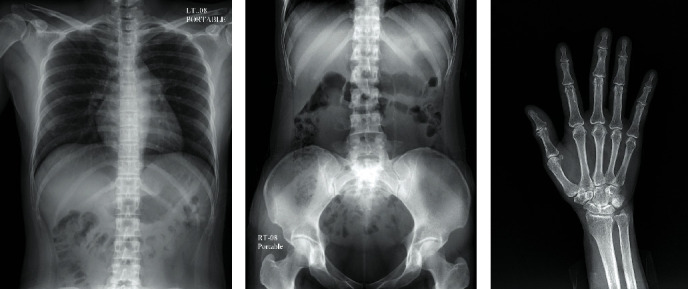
Sample radiographic images of the chest, the abdomen, and the right hand of the volunteers from the BodiiRay system.
